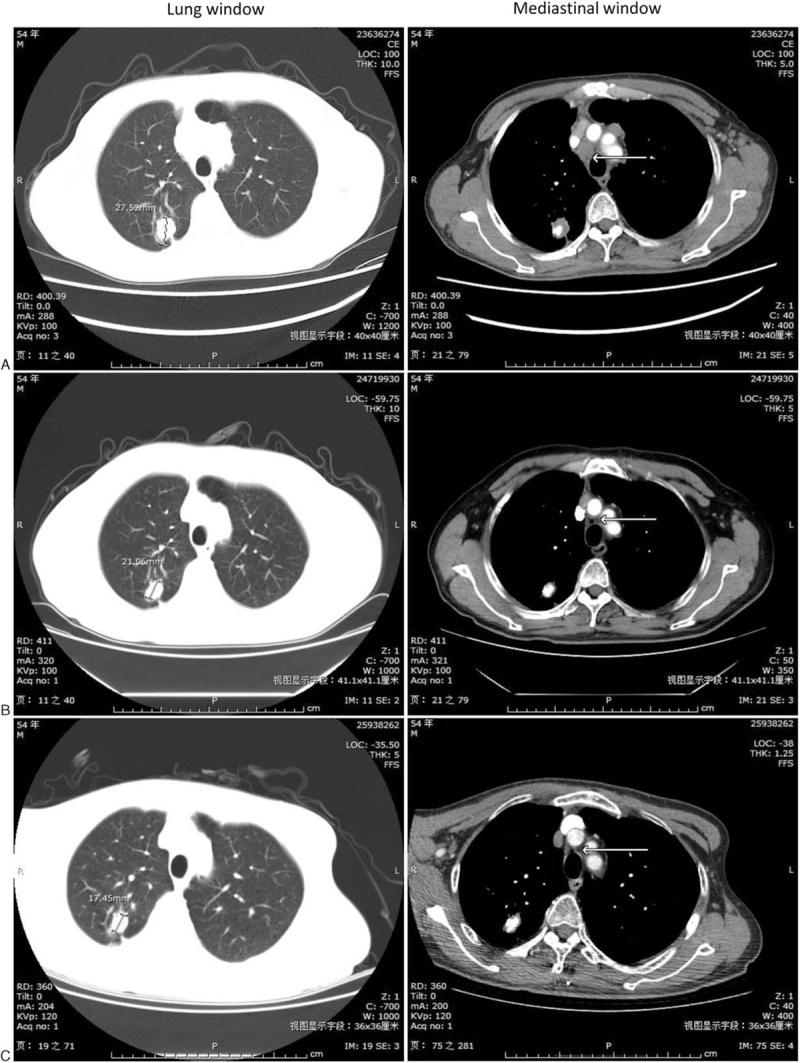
Image of chest computed tomography. (A) Before chemotherapy (November 2014); (B) After chemotherapy of pemetrexed/cisplatin for 6 cycles (April 2015); (C) After radiotherapy combined with chemotherapy of pemetrexed for 3 cycles (September 2015).
